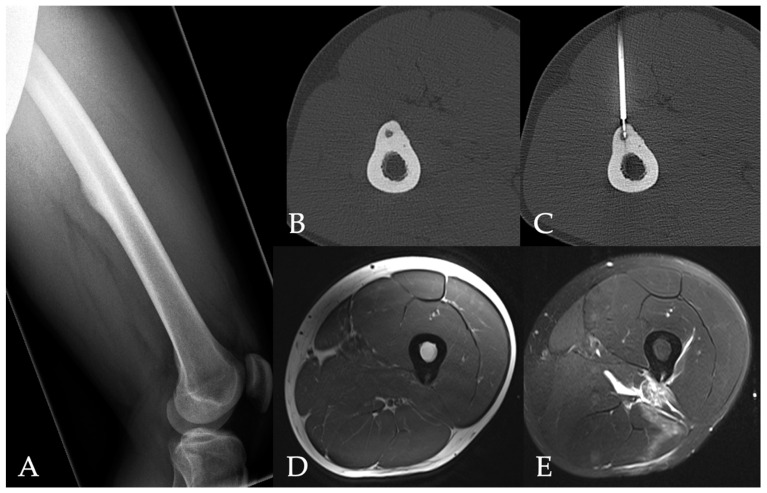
27-year-old male patient with an osteoid osteoma in the femur (A). Peri-interventional CT showing the lesion before and during radiofrequency ablation (B,C). Post-interventional MRI shows post-procedural changes including edema in the soft tissue without damage of the bone structure (D [Axial T1-weighted image], E [axial T2-weighted fat-saturated image]).
